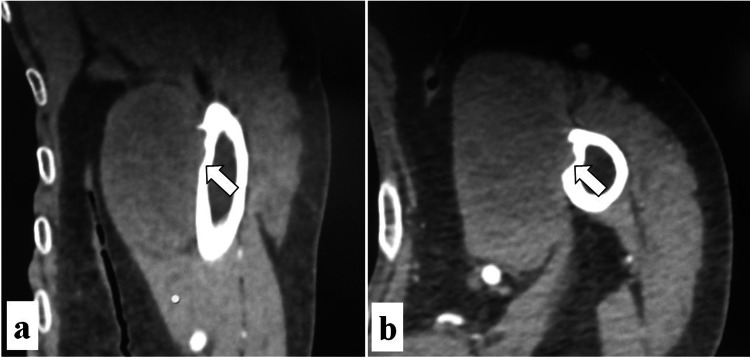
Computed tomography images of the left upper arm. (a) Coronal and (b) axial images showing the tumor partially encroaching into the cortical bone on the medial side of the humerus (white arrows).
